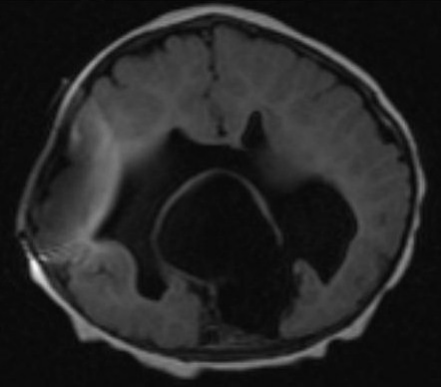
MRI of brain after Ventriculoperitoneal shunt placement; Single monoventricle with Hydrocephalus, partially fused frontal interhemispheric fissure consistent with semilobar HPE.
